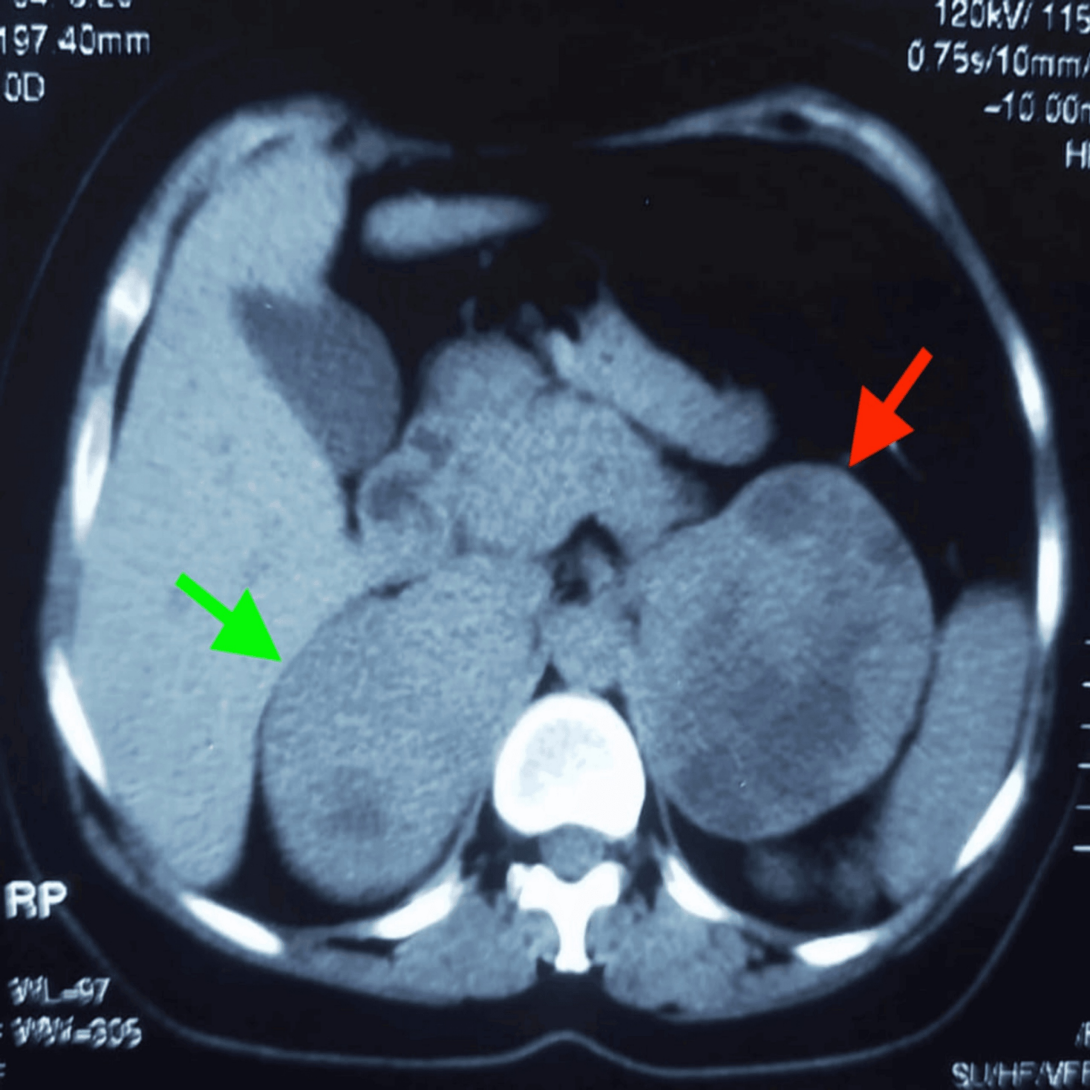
Axial contrast-enhanced CT Scan The scan shows bilateral adrenal masses consistent with pheochromocytomas. The right adrenal mass (green arrow) appears well-defined and heterogeneously enhancing, while the left adrenal mass (red arrow) shows similar enhancement characteristics. No evidence of local invasion or distant metastasis is seen.
